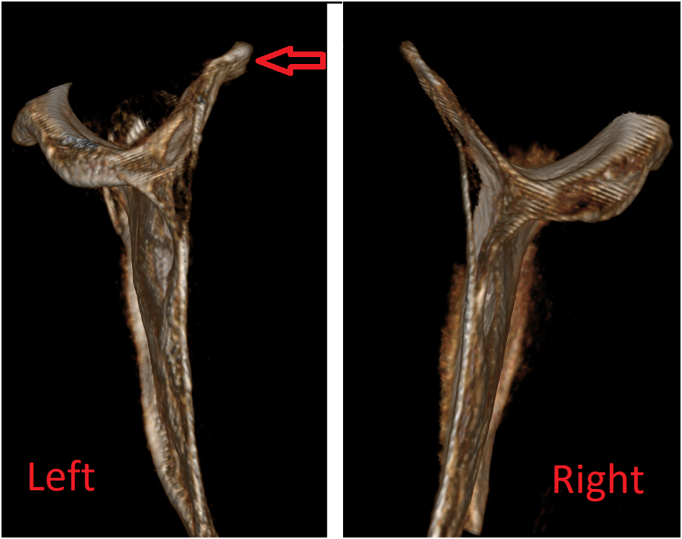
Preoperative CT Scan of Left and Right Scapulas-Note the deformity of the left scapular (Red Arrow)
